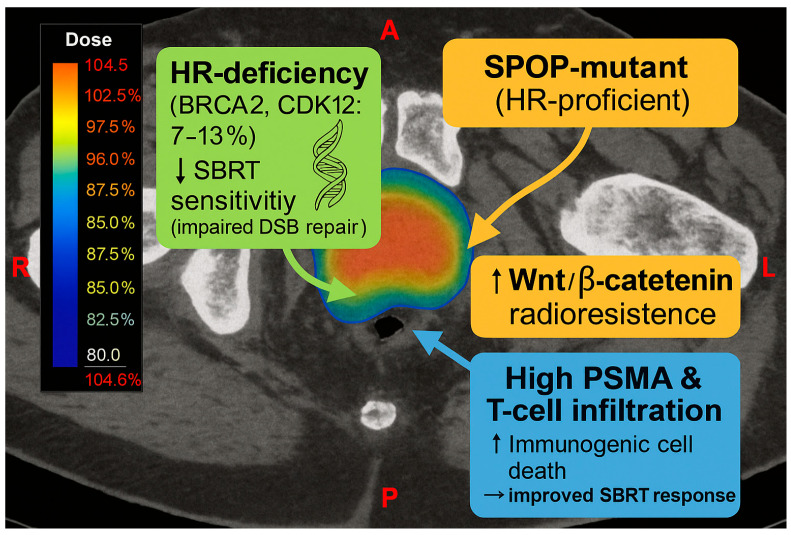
Axial CT slice from a prostate SBRT treatment plan. Tumor subregions demonstrate distinct radiogenomic and immunologic phenotypes. Green: HR-deficient lesions with impaired DSB repair and heightened SBRT sensitivity; Orange: *SPOP*-mutant, HR-proficient regions with Wnt/β-catenin activation and increased radioresistance; Blue: PSMA-high, T-cell–rich areas exhibiting improved SBRT response via immunogenic cell death.
